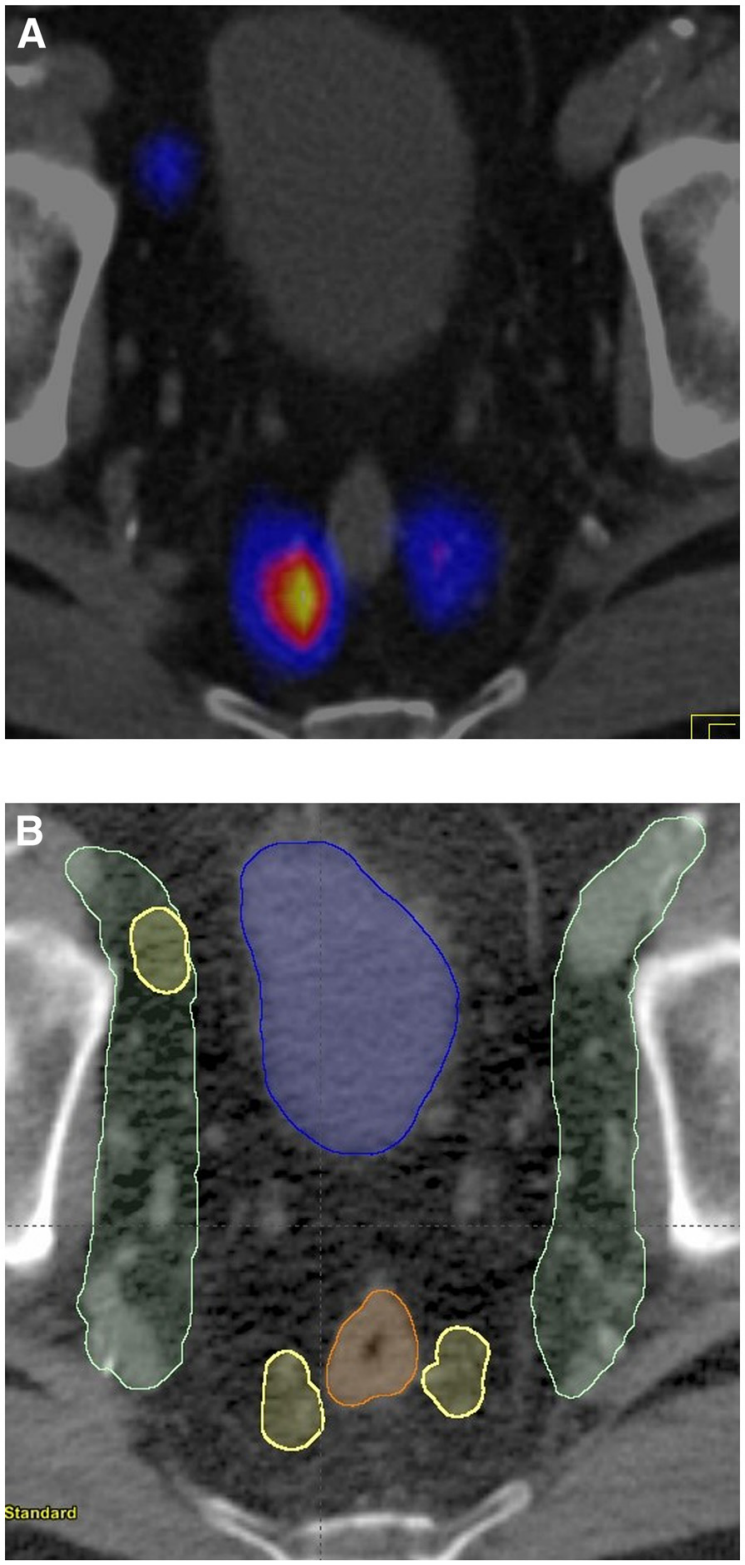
(A) Fusion image of SPECT and planning CT (patient no. 6) showing a transverse section of two pararectal and a right external iliac SN. (B) Axial view of the standard nodal clinical target volume (CTV) (green) and the pararectal SNs (yellow).
